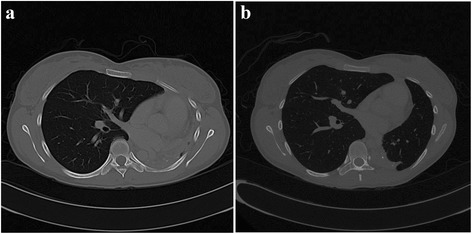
Axial view CT Thorax pre- and post-intervention. a CT Thorax showed mediastinal shift to the left. b CT Thorax at 36 weeks following intervention showed normal location of the mediastinum
